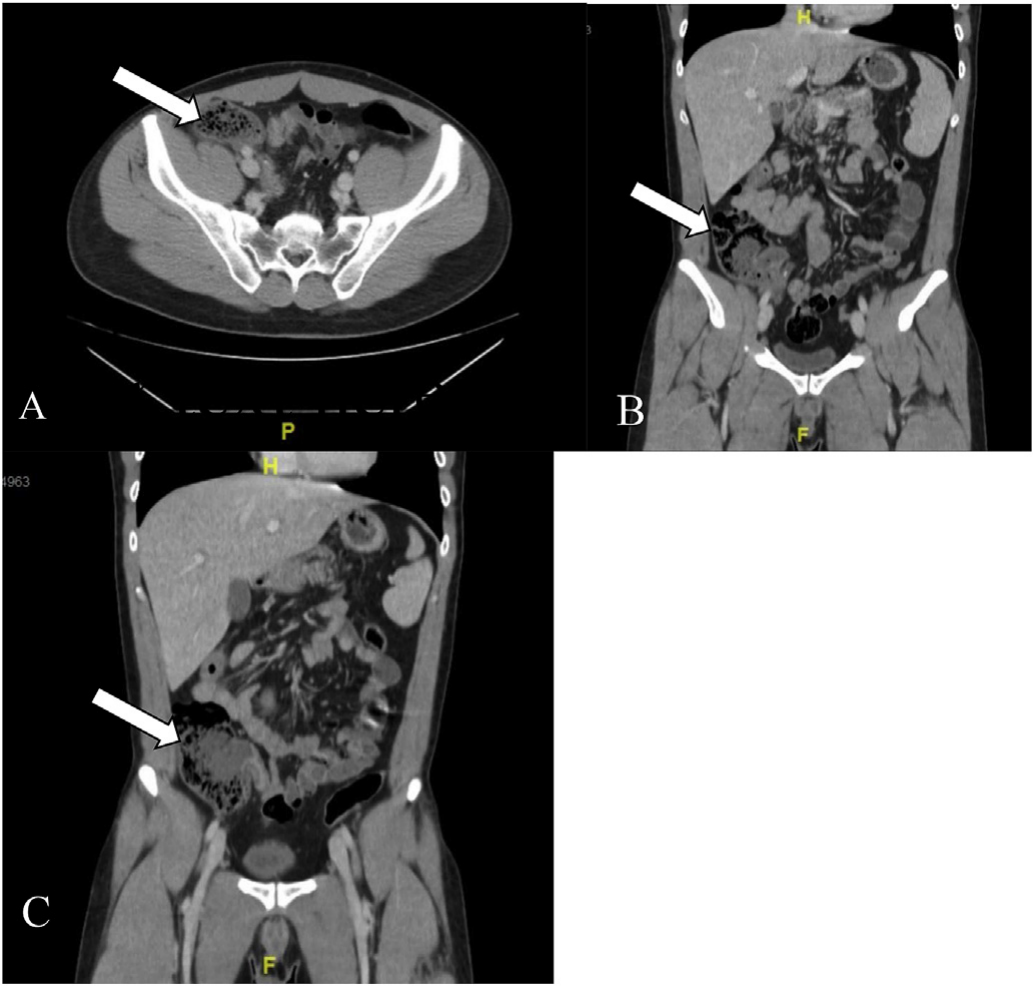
A. Acute appendicitis, Right lower quadrant, Abdomen/Pelvis CT scan. B- Acute appendicitis, RLQ, CT scan of abdomen/pelvis, coronal view; seen is an appendicolith at the base of appendix. C- Acute appendicitis, Right lower quadrant, base of cecum is seen and fluid filled 10–11 mm dilated appendix, CT scan of abdomen/pelvis, Coronal view.
